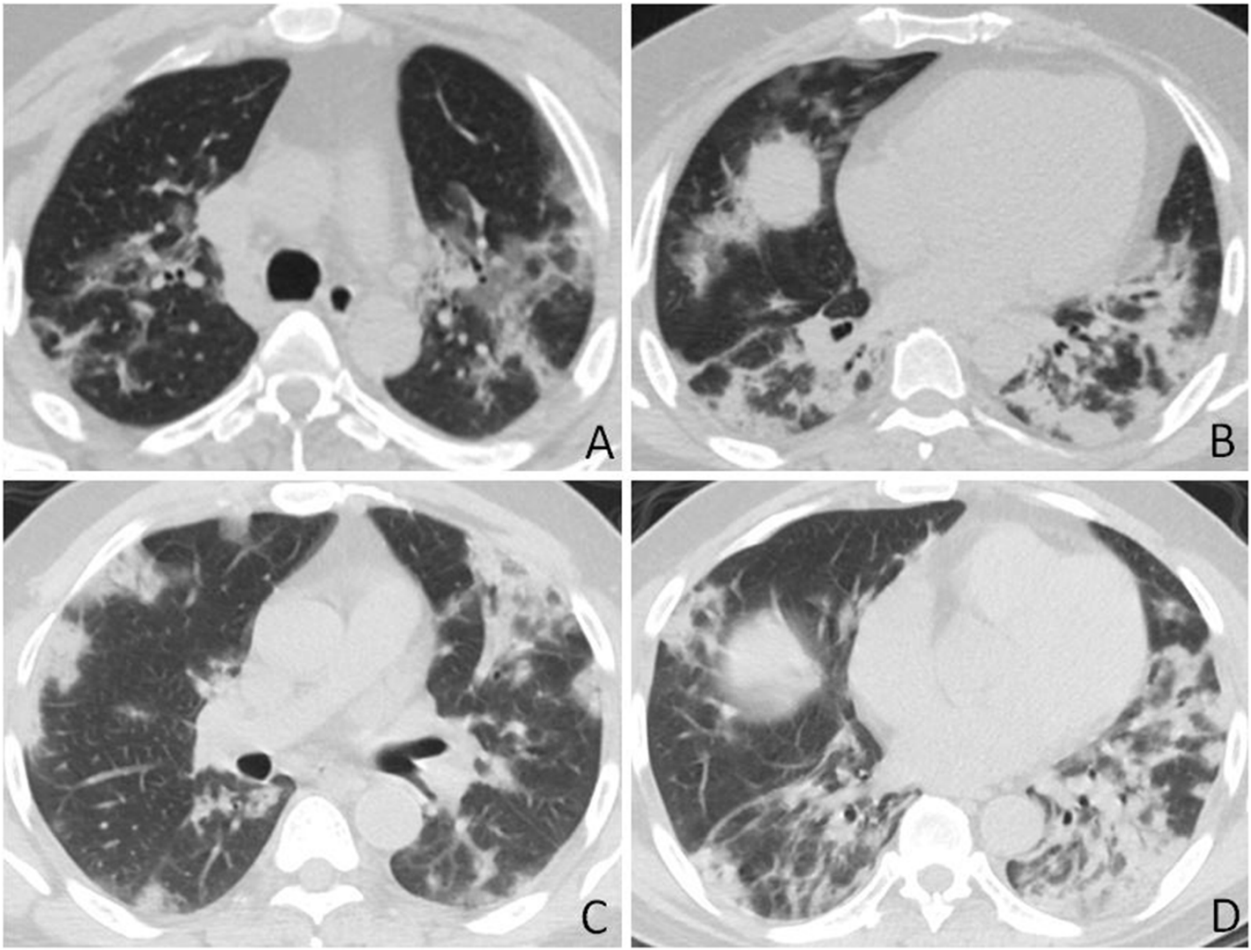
COVID-19 and influenza A pneumonia misdiagnosed as COVID-19. a and b Axial CT images obtained from a 43-year-old woman with positive PCR test for COVID-19 show peripherally located multifocal areas of poorly defined focal consolidation, small areas of GGO, and bronchial wall thickening. c and d Axial CT images from a 61-year-old man with positive PCR test for influenza A (H1N1) show similar features as a and b with predominant consolidations
